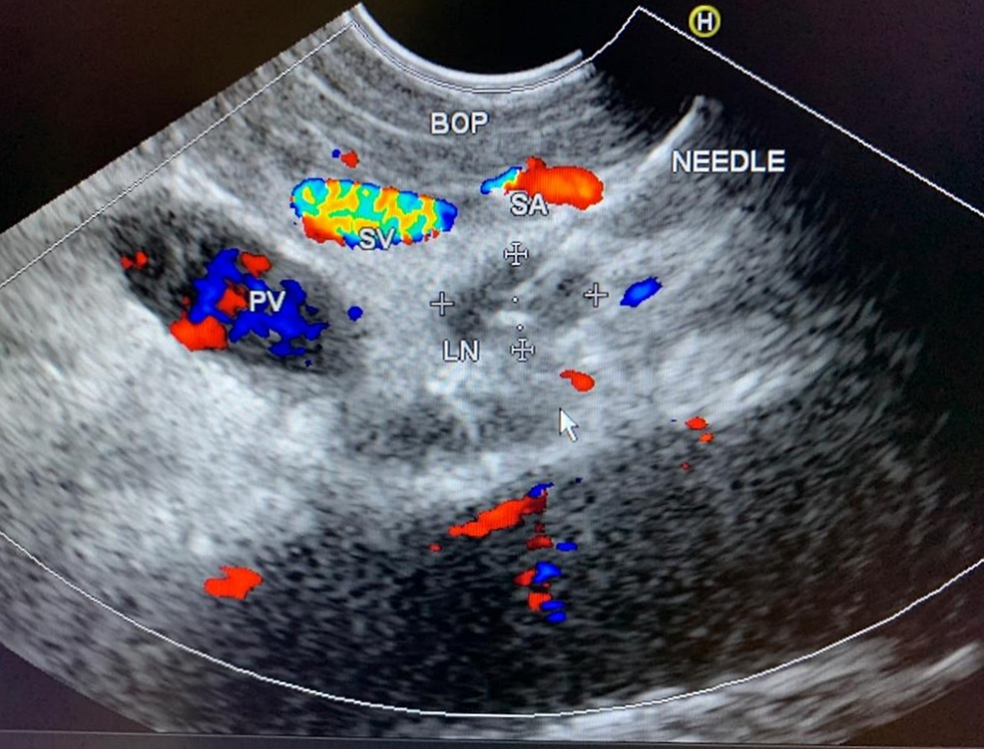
Endoscopic ultrasound. PV, portal vein; BOP, body of pancreas; LN, lymph node; SV, splenic vein; SA, splenic artery
